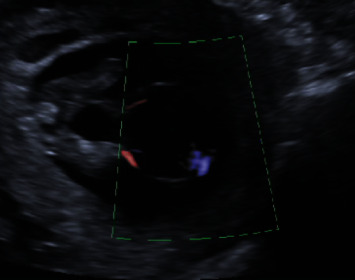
Allantoic cyst during ultrasound examination at the 13th week of gestation. Note its position within the umbilical cord and adjacent to the abdominal wall. It is avascular, in communication with the bladder and embraced by the two umbilical arteries.
